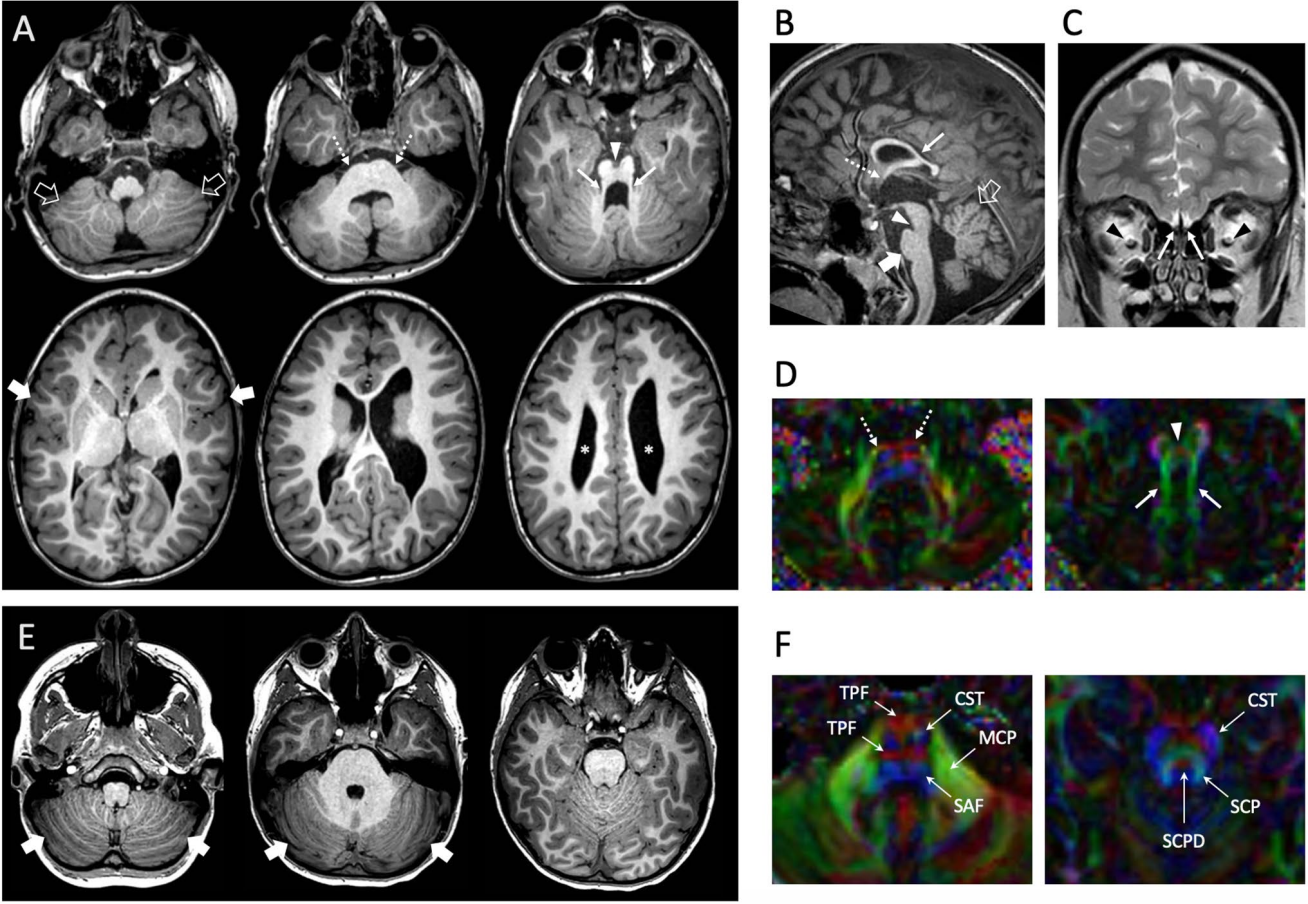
Neuroimaging features of the NAV2-related neurodevelopmental disorder in the proband. Brain MRI of the patient performed at 6 years of age (A–D) and of an age-matched control subject (E–F). A) Axial reformatted 3D T1-weighted images reveal abnormal cerebellar foliation fissuration, more pronounced at the level of the inferior cerebellar hemispheres (empty arrows). The pons is flattened and slightly asymmetric (dotted arrows). The superior cerebellar peduncles are thinned and splayed (arrows) with associated narrowing of the isthmic region (arrowhead) leading to a molar tooth-like appearance of the midbrain. Note the diffuse cortical dysgyria, with prevalent insular involvement (thick arrows), and the asymmetric enlargement of the lateral ventricles (asterisks). (B) Sagittal reformatted 3D T1-weighted image shows the hypoplasia and dysplasia with mild upward rotation of the cerebellar vermis, and prevalent involvement of the anterior and superior posterior lobes (empty arrows). There are also corpus callosum hypodysgenesis (arrow), agenesis of the anterior commissure (dotted arrow), narrow isthmus (arrowhead), and small pons (thick arrow). Note the small optic nerve chiasm. (C) Coronal T2-weighted image demonstrates the agenesis of the olfactory bulbs (arrows) and mild hypoplasia of the optic nerves (arrowheads). (D) Diffusion tensor imaging, axial color-coded fractional anisotropy (FA) maps reveal small asymmetric corticospinal tracts at the level of the pons (dotted arrows) and horizontal course of the superior cerebellar peduncles (arrows) with preservation of their decussation at the midbrain level (arrowhead). (E) Axial reformatted 3D T1-weighted images show the normal size and morphology of the cerebellar hemispheres. The cerebellar folia run parallel to the calvarium (onion-like configuration; thick arrows). (F) Axial color-coded FA maps, magnified view at 2 brainstem levels (middle pons and middle midbrain). Conventional color scheme: blue (inferior-superior), green (anteroposterior), and red (left–right). CST, corticospinal tract; MCP, middle cerebellar peduncles; SCP, superior cerebellar peduncles; SCPD, superior cerebellar peduncles’ decussation; SAF, somatosensory ascending fibers; TPF, transverse pontocerebellar fibers
